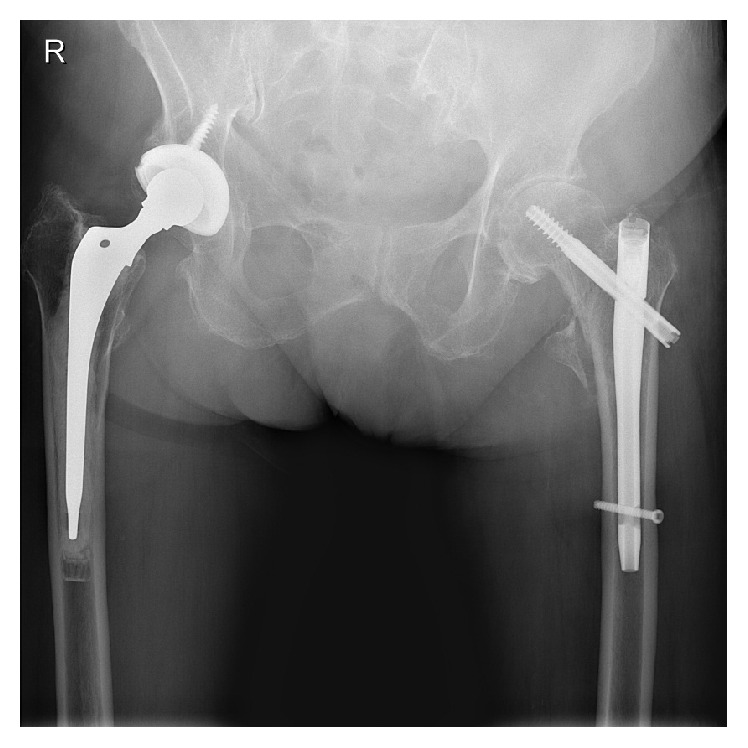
Anterior-posterior X-ray 6 weeks after operation, showing unchanged position and signs of consolidation.
